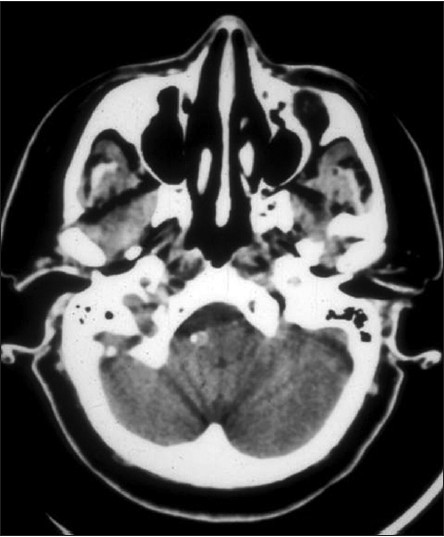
Patient number 3. MRI, sagittal view of medulla. Note right vertebral artery compressing anterolateral medulla
